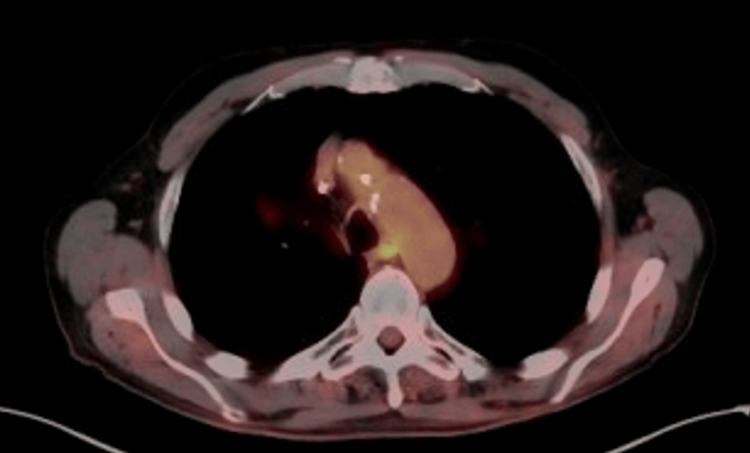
Axial PET/CT of the chest with FDG-18 20 months post-chemoradiation and 15 months post-immunotherapy does not indicate the presence of a hypermetabolic mass
